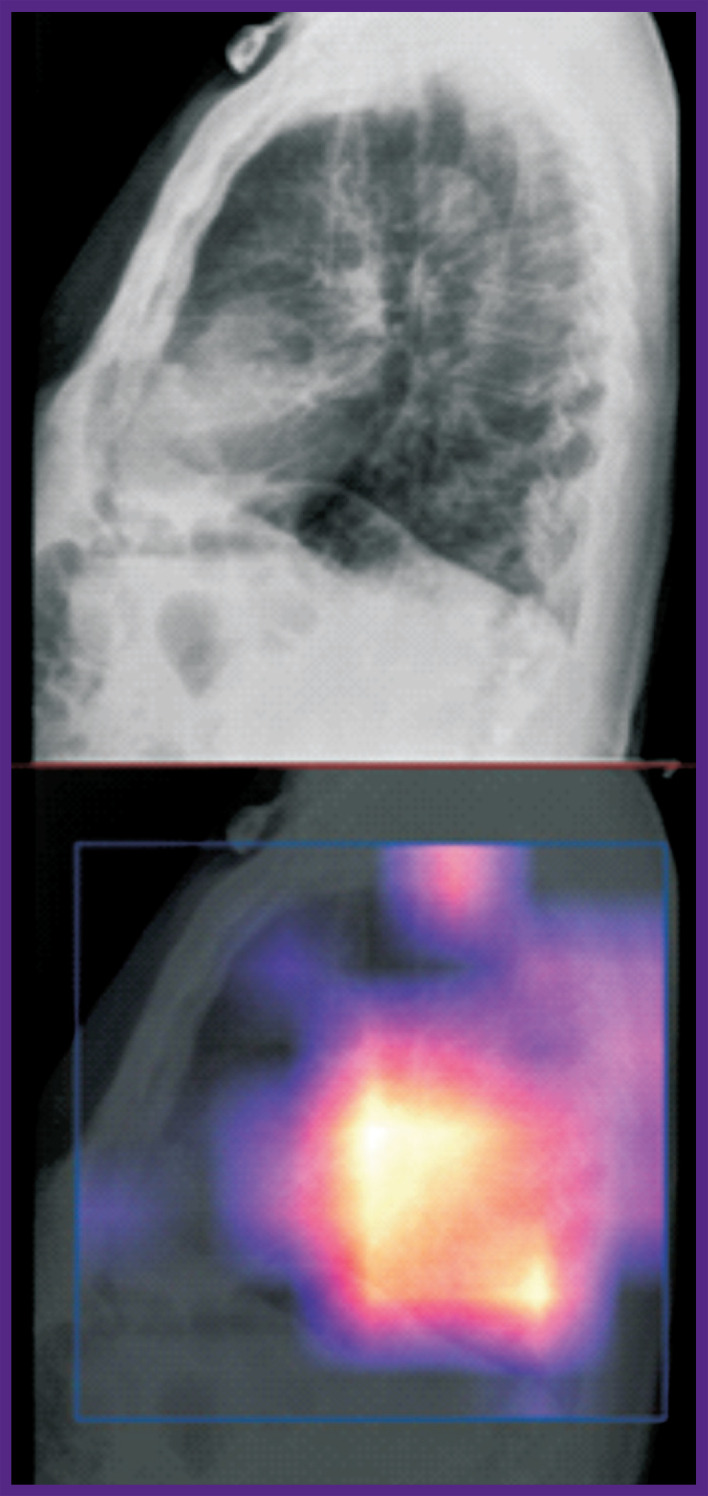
An example of an SaMD-AI technical flaw (2.2, see the Table) using evidence from chest radiography
